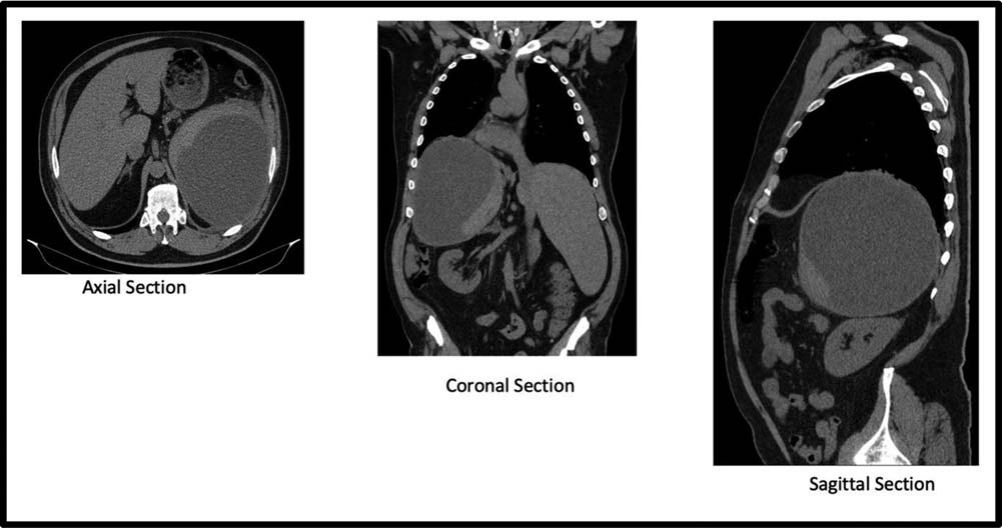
Thoracoabdominal computed tomography sections showing splenomegaly of 20 X16X18 cm, with a subcapsular hypodense collection, homogeneous fluid densities, mass effect on the left hemidiaphragm, compressive atelectasis, and focal pulmonary consolidation.
